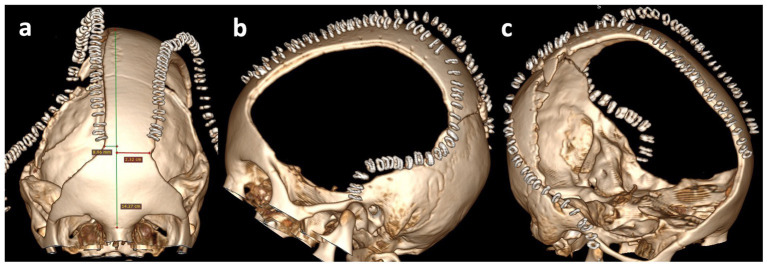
3-D reconstruction of postoperative head CT scan of a patient who underwent bilateral decompressive craniectomies for severe traumatic brain injury: (a) right-sided craniectomy’s medial edge is located very close to the anatomical midline (< 1 cm), while left-sided craniectomy is >2 cm from the midline, (b) left-sided craniectomy, (c) right-sided craniectomy.
